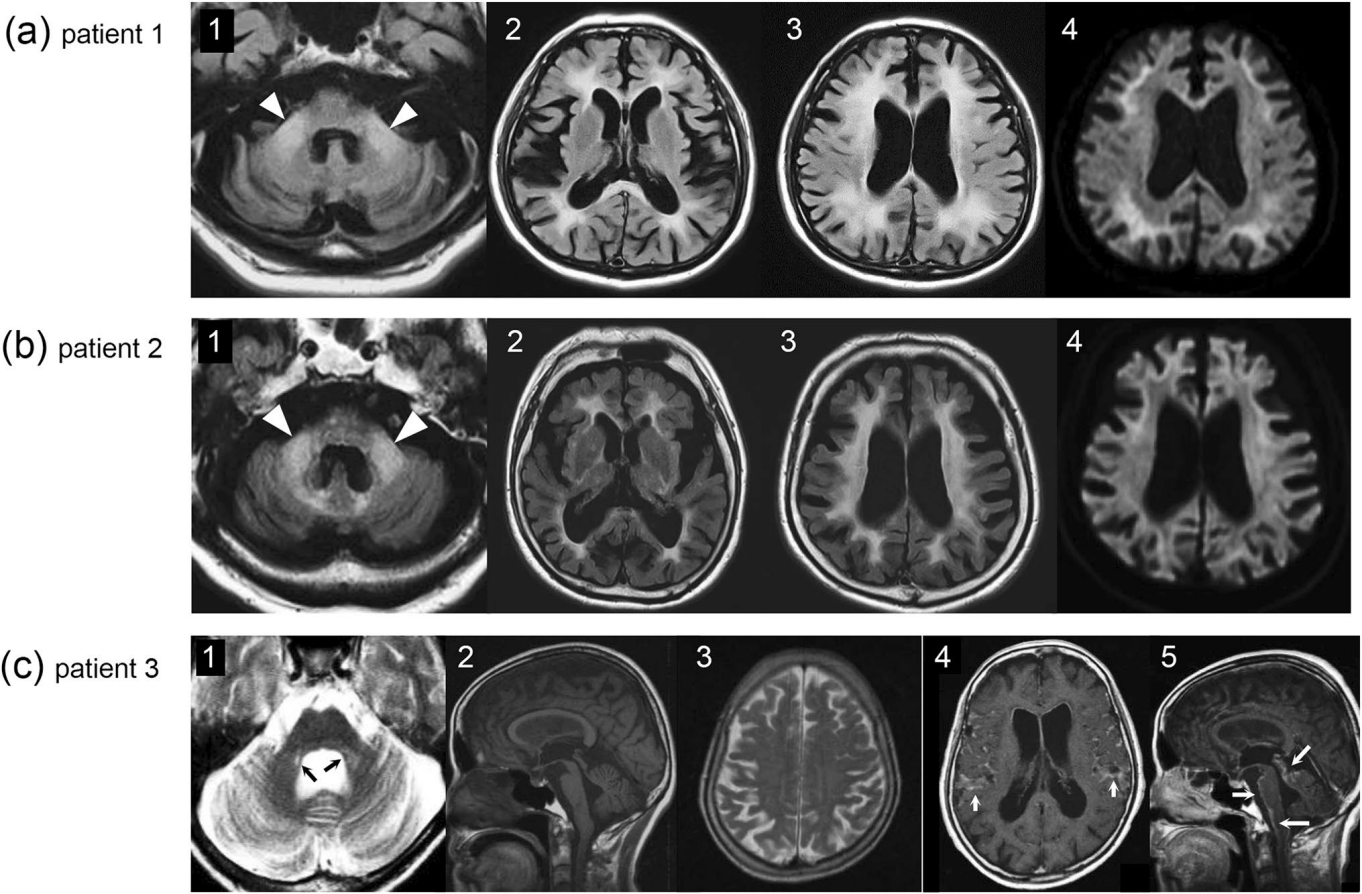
Magnetic resonance imaging (MRI) findings. Brain MRI of (a, 1–4) patient 1 at the age of 73 years, (b, 1–4) patient 2 at the age of 83 years, (c, 1 and 2) patient 3 at the age of 68 years, and (c, 3 and 4) patient 3 at the age of 69 years. Axial fluid-attenuated inversion recovery images of patients 1 and 2 showing high-intensity lesions in the (a, 1; b, 1) bilateral middle cerebellar peduncles (‘MCP sign’) as indicated by white arrowheads, (a, 2; a, 3) corpus callosum and (a, 3; b, 3) cerebral white matter. Moreover, a linear high signal intensity in the corticomedullary junction on diffusion-weighted imaging can be observed (a, 4; b, 4). Axial and sagittal images from patient 3 show (c, 1–3) atrophy of the pons, cerebellum, and cerebral cortex with a (c, 1) faint MCP sign (black arrows). At the age of 69, brain gadolinium-enhanced MRI showed (c, 4; c, 5) leptomeningeal enhancement of the meninges around the brainstem and cerebellum (white arrows)
